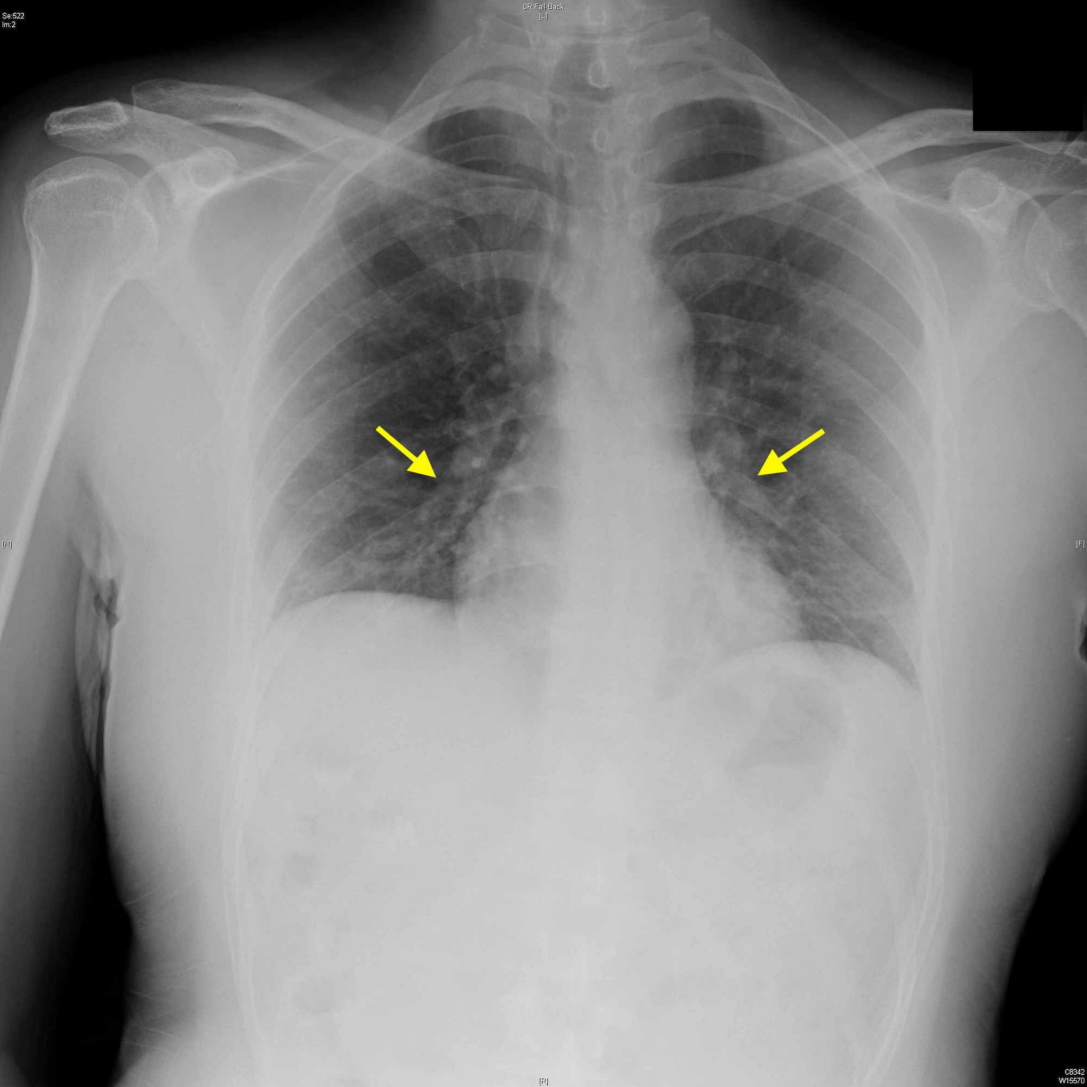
Chest radiograph demonstrating bilateral opacities associated with multifocal pneumonia (arrows)
